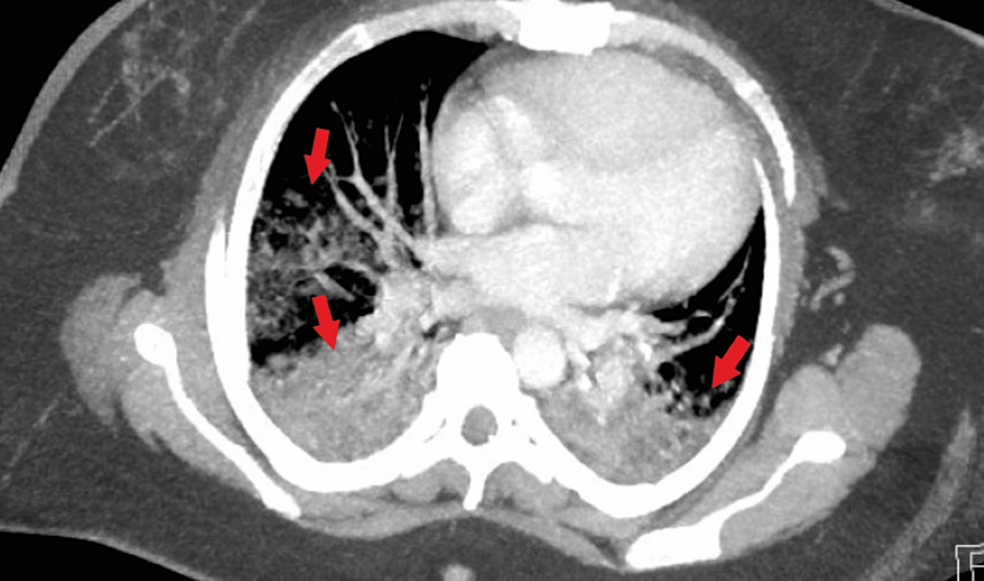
Computed tomography angiogram of the chest revealing extensive bilateral alveolar consolidation and ground-glass opacification.
